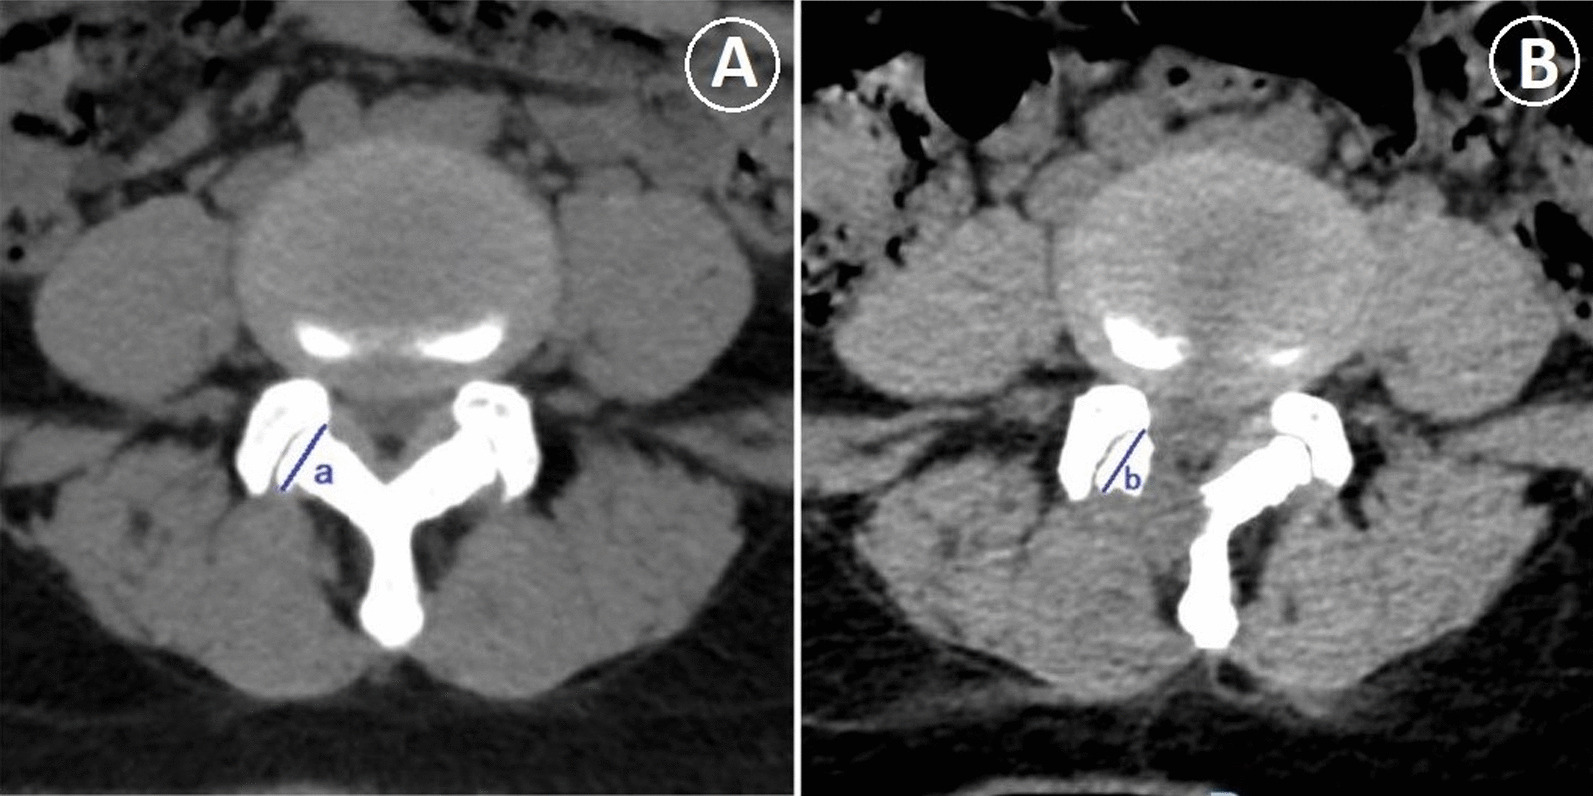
A, B The postoperative facet preservation rate was assessed by measuring the axial articular surface length of the preoperative and postoperative responsible segments on lumbar horizontal CT scan image. Postoperative facet preservation rate = b/a × 100%
